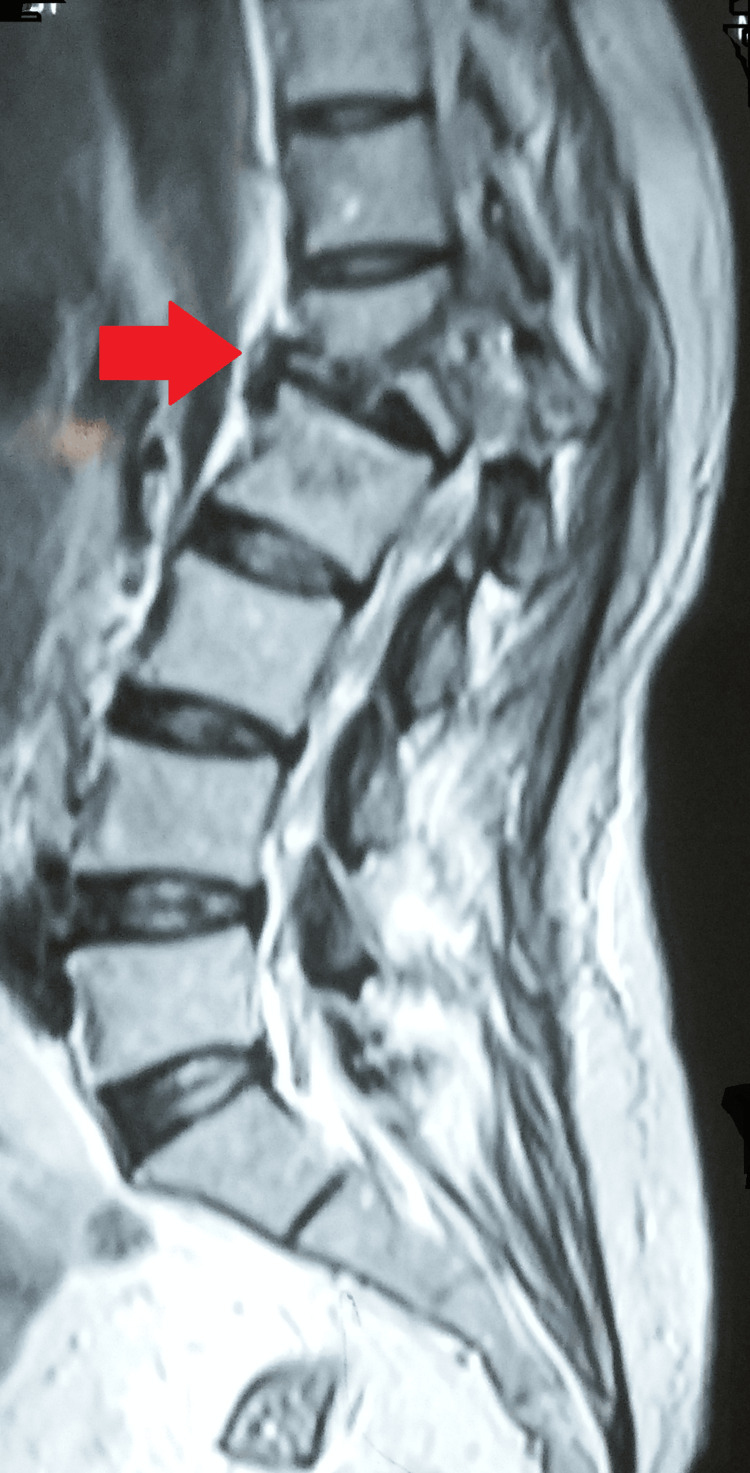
MRI of the dorso-lumbar spine showing a partial collapse of the D12 vertebral body and a significant collapse of the L1 vertebral body with a loss of the D12-L1 height
